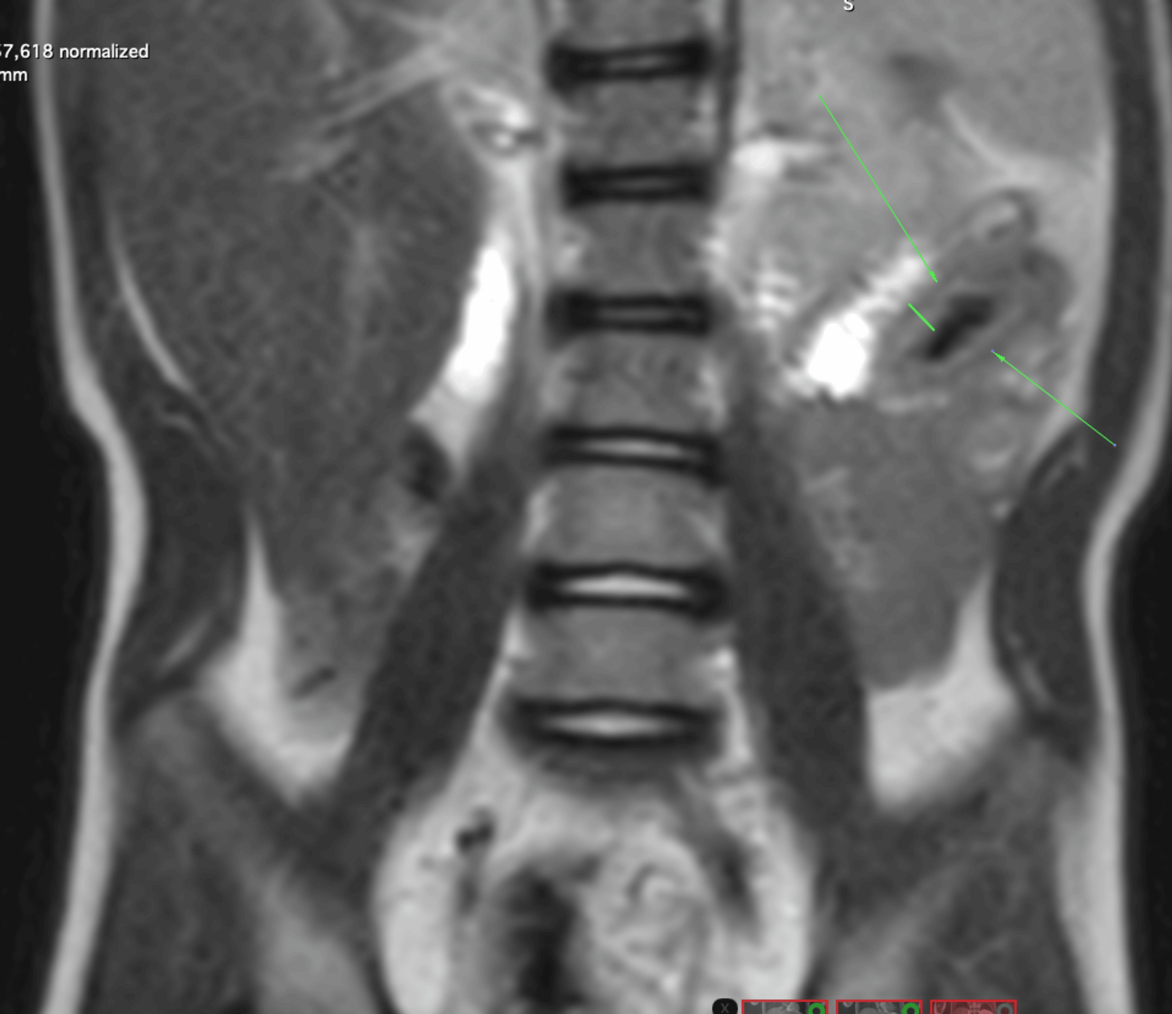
Coronal abdominal magnetic resonance imaging demonstrating bowel wall thickening Coronal T2-weighted magnetic resonance imaging of the abdomen demonstrating segmental bowel wall thickening with a cobblestone appearance, consistent with inflammatory bowel disease-related changes (arrows).
